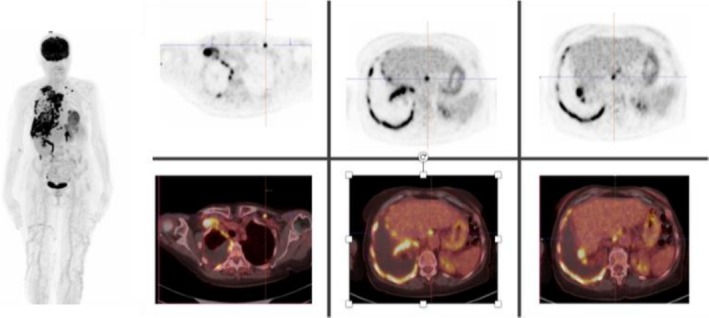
18F‐FDG PET/CT images showing multiple areas of pathological uptake. The maximum intensity projection (left) highlights widespread hypermetabolic lesions. Axial PET images (top row) and fused PET/CT images (bottom row) demonstrate increased FDG uptake in various sites.
